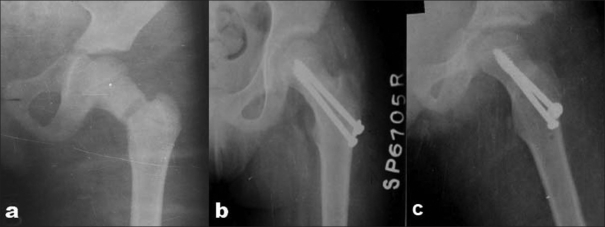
(a) X-ray hip joint (anteroposterior view) showing displaced femoral neck fracture (type II) in a 9-year-old boy, presented after 4 days of injury. (b,c) Follow-up radiographs (anteroposterior and lateral view) after 30 months showing union without any signs of AVN
